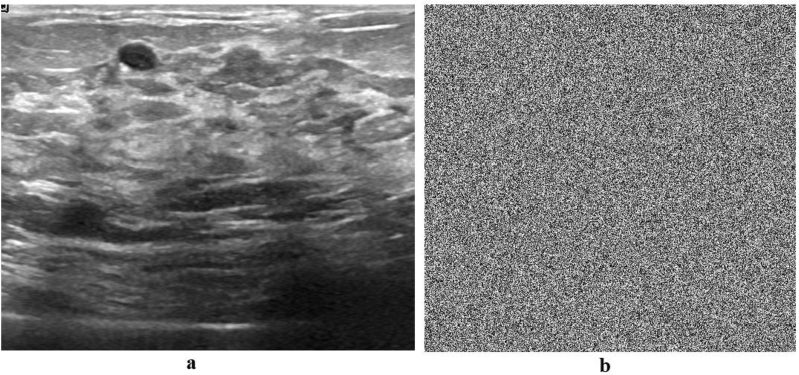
Plain and encrypted ultrasound image.
